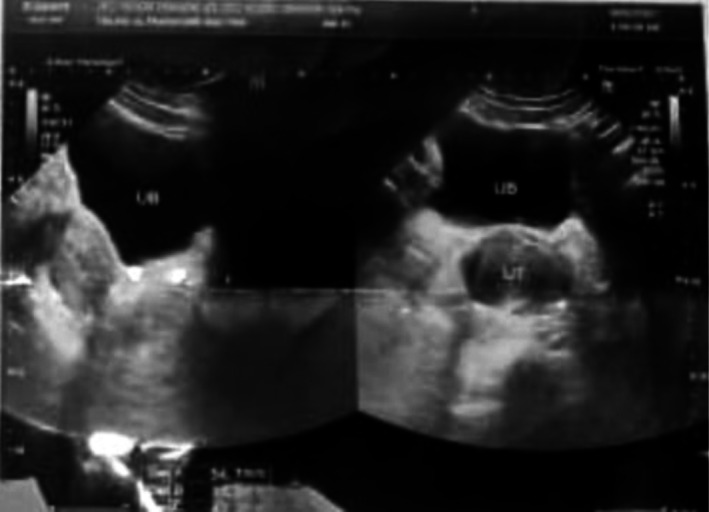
Transabdominal pelvic ultrasound showing hematometra in a 16‐year‐old female with cervicovaginal agenesis. The sagittal and transverse views demonstrate a distended uterine cavity (UT) filled with homogeneous hypoechoic fluid, consistent with hematometra. The urinary bladder (UB) appears anterior to the uterus. The cervical canal and vaginal canal are not visualized, supporting the diagnosis of cervicovaginal agenesis as the underlying cause of obstructed menstrual outflow.
